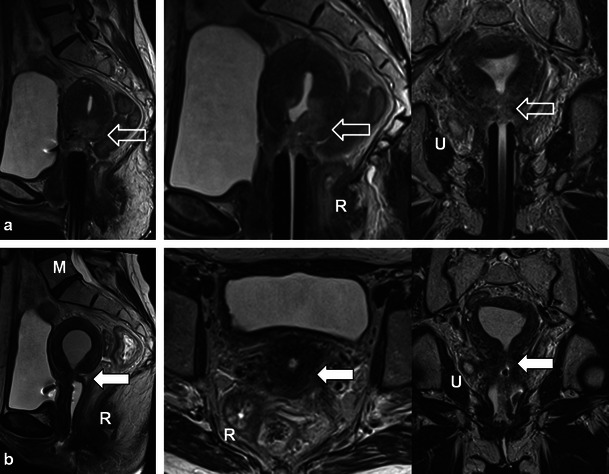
A 46-year-old woman with locally advanced (Stage IIIB) cervical squamous cell carcinoma (*open white arrows*) with right ureteral involvement (*U*) depicted on treatment planning pelvic MRI with sagittal and coronal oblique T2 TSE sequences. Note the tumour is of intermediate T2 signal intensity (*open white arrows*) and that the brachytherapy device is appropriately positioned at the level of the tumour. Follow-up MRI demonstrates interval reduction of tumour size and T2 signal intensity with focal atrophy at the cervical os (*closed white arrow*) with progression of now moderate hydrometra on sagittal, axial and coronal oblique T2 TSE sequences. The findings are consistent with treatment response and post therapeutic cervical stenosis which is a common finding after RT for cervical carcinoma. Also note radiation proctitis (*R*) in (a) which resolves after therapy in (b) and fatty marrow replacement (*M*) in b after therapy
